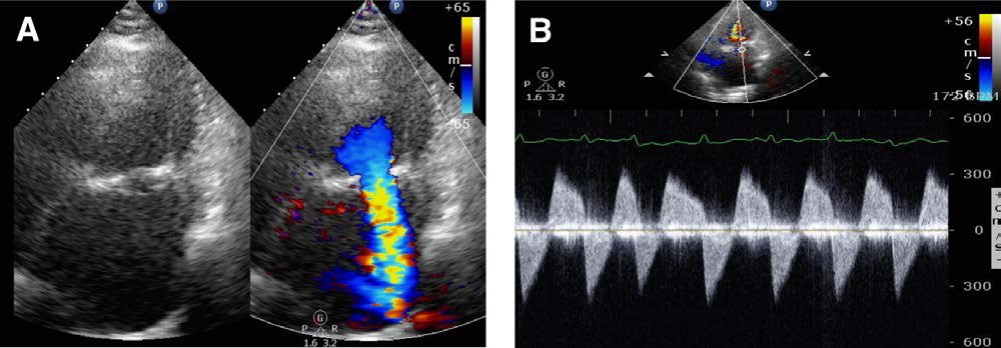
(A) In the TTE 4-chamber view, color Doppler flow indicated severe regurgitation due to prosthetic mitral valve dysfunction. (B) CW Doppler revealed a high-speed regurgitant flow into the left atrium. CW = continuous-wave, TTE = transthoracic echocardiography.
